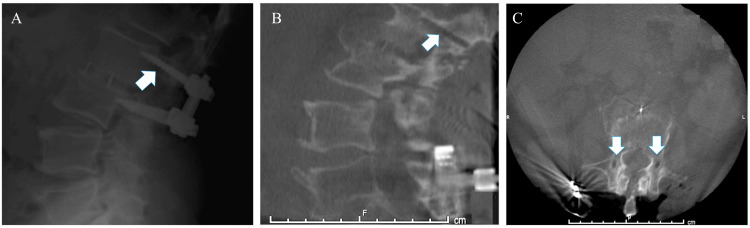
Preoperative standing upright lateral X-ray shows cortical screws with hypodensity surrounding the screws superiorly concerning for pseudoarthrosis (A). A preoperative lumbar CT scan shows the caudal-to-cranial (B) and medial-to-lateral (C) path of removed cortical screws in a sagittal and axial frame.
